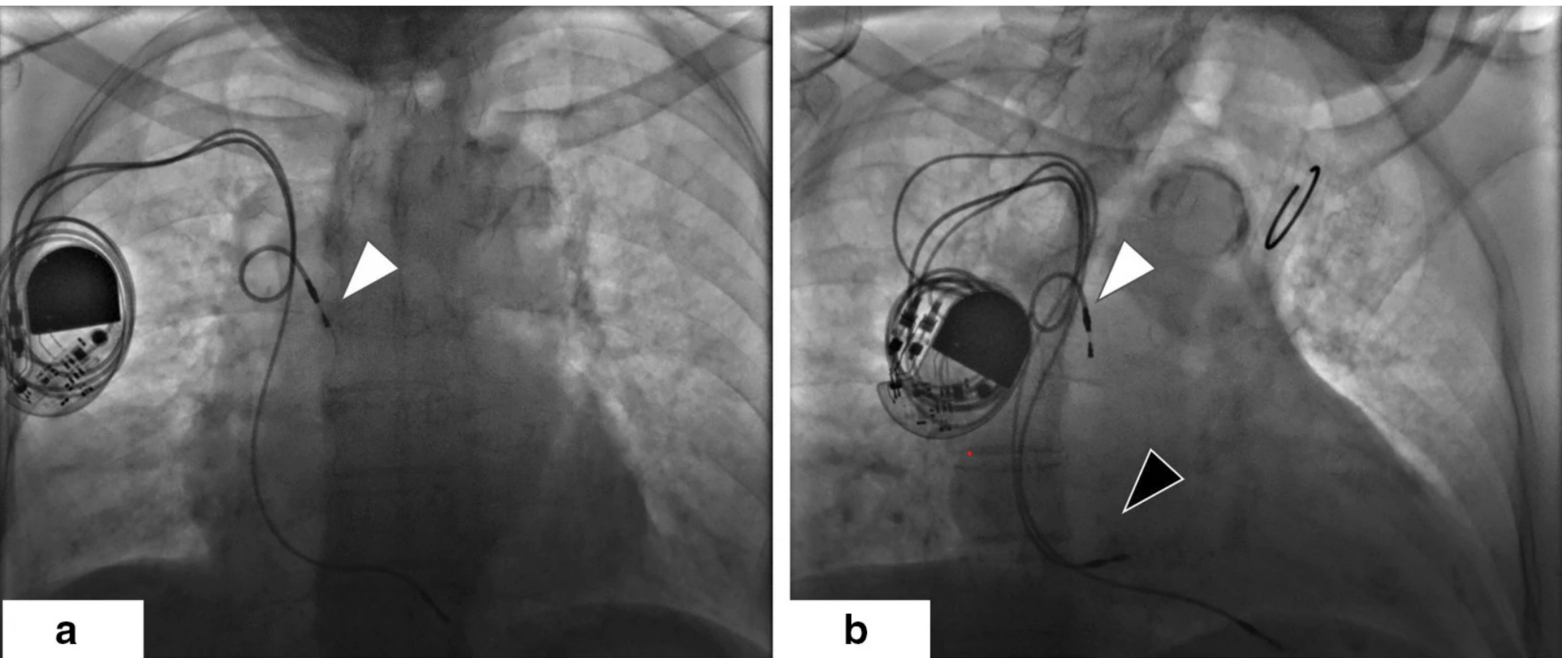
Anteroposterior projected radiograph of the patient before (a)
